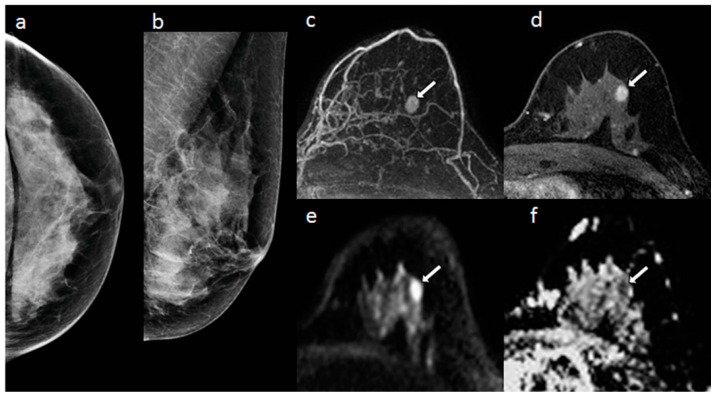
A 58-year old woman with dense breasts and invasive ductal carcinoma. (a) X-ray mammogram CC view and (b) MLO view, (c) DCE maximum intensity projection, (d) axial T_1_-weighted fat saturated DCE-MRI, (e) axial DWI, and (f) ADC map [5]. “Reprinted with permission from Ref. [5]. 2011, springer”. More details on “Copyright and Licensing” are available via the following link: https://link.springer.com/article/10.1007/s00330-010-1890-8. White arrows indicated lesion in the images.
